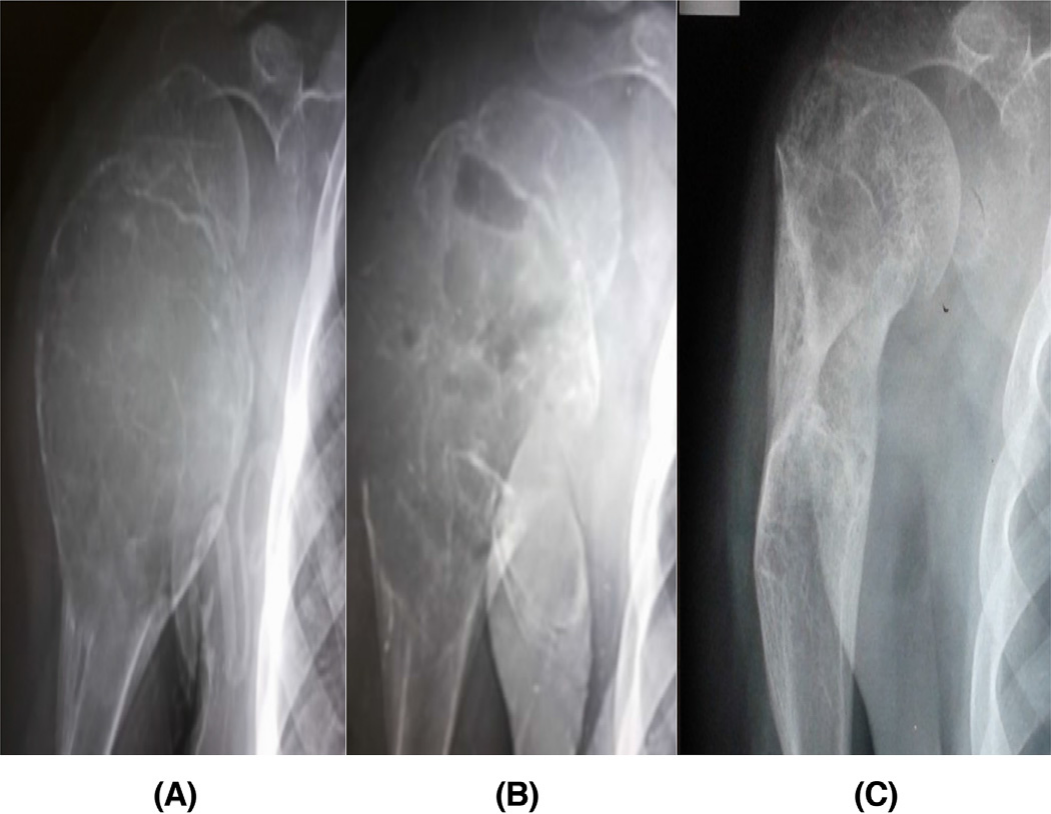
Serial plain radiographs (A) 11 years old patient with ABC lesion in the proximal humerus (case 9), (B) 4 weeks follow-up after endoscopic curettage without grafting showed new bone formation, and (C) complete healing and remodeling after 24 months.
